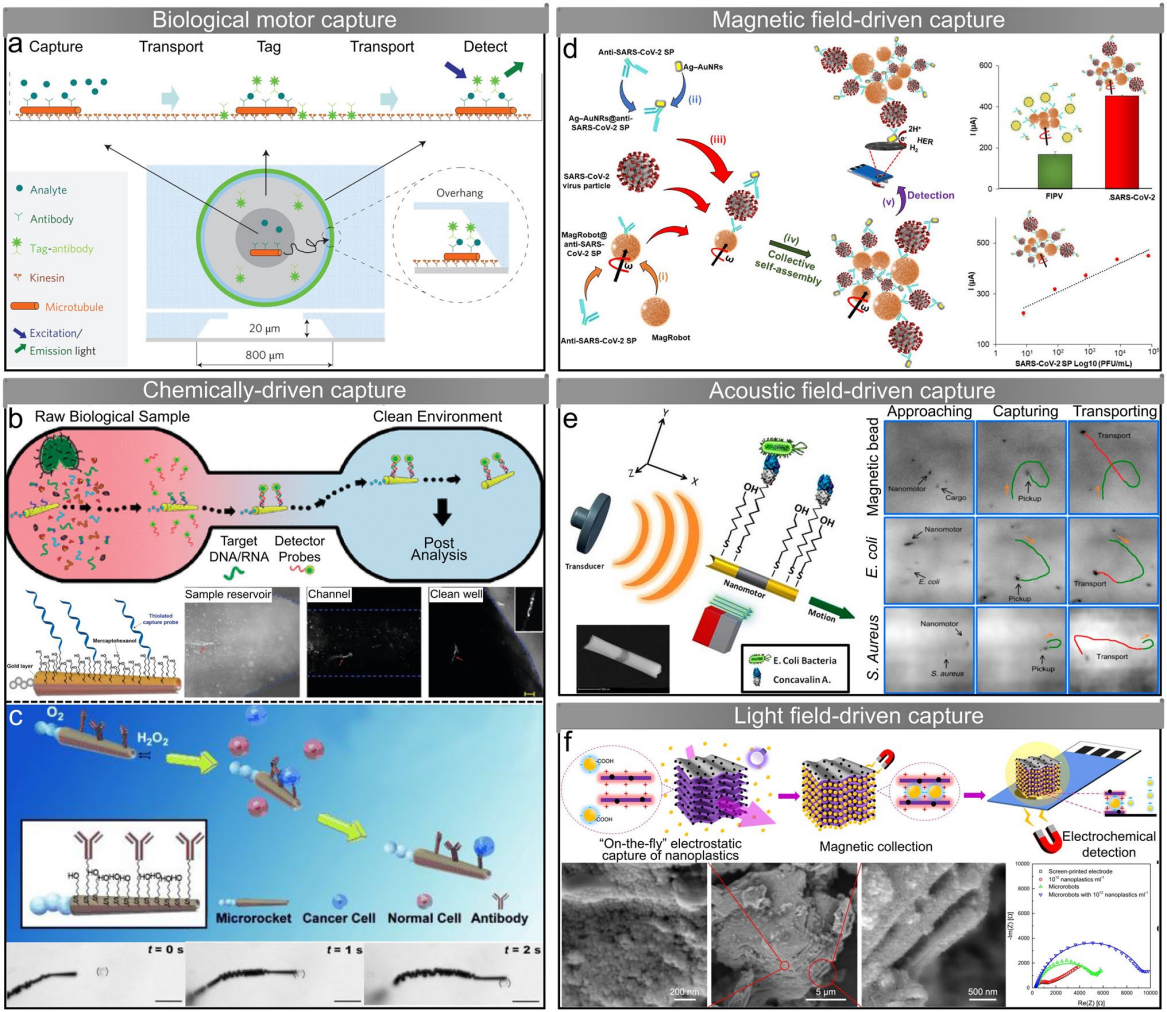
Micro/nanorobots selectively capture and transport targets for sensing. a A “smart dust” microdevice based on biomolecular motors for capturing and transporting target analytes [119]. Copyright (2009) Springer Nature. b Bubble-propelled catalytic Ti/Ni/Au/Pt microtubes for isolating nucleic acid from raw biological samples. The optical images show the microtube capturing target DNA from a sample reservoir and transporting it through a 6 mm-long channel into a clear well [122]. Copyright (2011) American Chemical Society. c The Ti/Fe/Au/Pt microtubes selectively capture target cancer cells through specific antigen recognition. The optical images show the microtube capturing and transporting a CEA+ pancreatic cancer cell in diluted human serum [123]. Copyright (2011) John Wiley and Sons. d Magnetic microrobots capture SARS-CoV-2 virus and Ag-AuNR tags and self-assemble into 3D-staggered chains through immuno-sandwich assay. Subsequent detection is achieved based on the hydrogen evolution reaction of Ag-AuNR tags [124]. Copyright (2022) Elsevier. e Ultrasound-powered magnetically guided Au-Ni-Au nanowires for selective capture and transport of bacteria. The optical images show capture and transport of a magnetic bead, *E. coli* bacteria, and *S. aureus* bacteria, respectively [125]. Copyright (2013) American Chemical Society. f Light-powered MXene-derived γ-Fe_2_O_3_/Pt/TiO_2_ microrobots for capture and detection of nanoplastics. The SEM images show microrobots with nanoplastics captured [126]. Copyright (2022) Springer Nature
